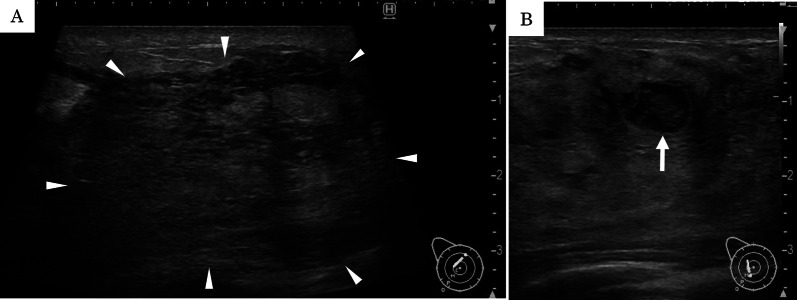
B-mode gray ultrasonography of the right breast. A Partially heterogeneous hypoechoic lesion (arrowhead). B Presence of a cyst suspected abscess (arrow)
